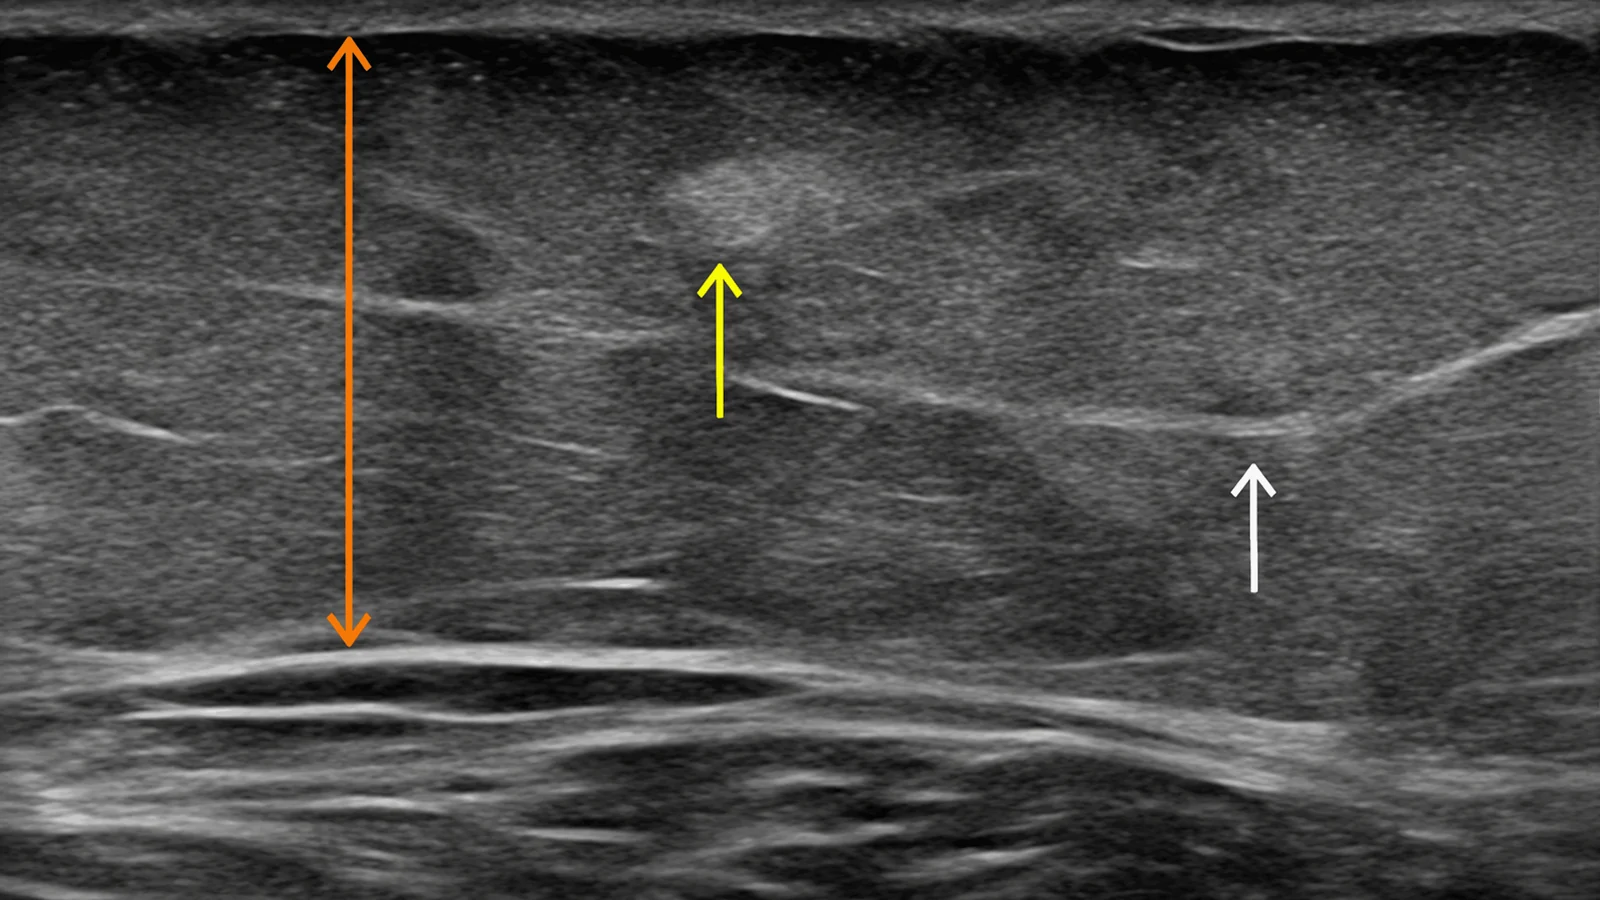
Ultrasound of a thigh nodule in a patient with lipedema Ultrasonographic image of a thigh nodule in a patient with lipedema (Lipedema Dermal and Hypodermal Classification 3) demonstrating thickened hypodermis (orange arrow), bulging deep hypodermis, disorganized superficial hypodermis, irregular septa (white arrow) predominantly in the superficial hypodermis, presence of a hyperechoic nodule (yellow arrow), asymmetric dermal thickening, and an irregular dermal-hypodermal junction.
